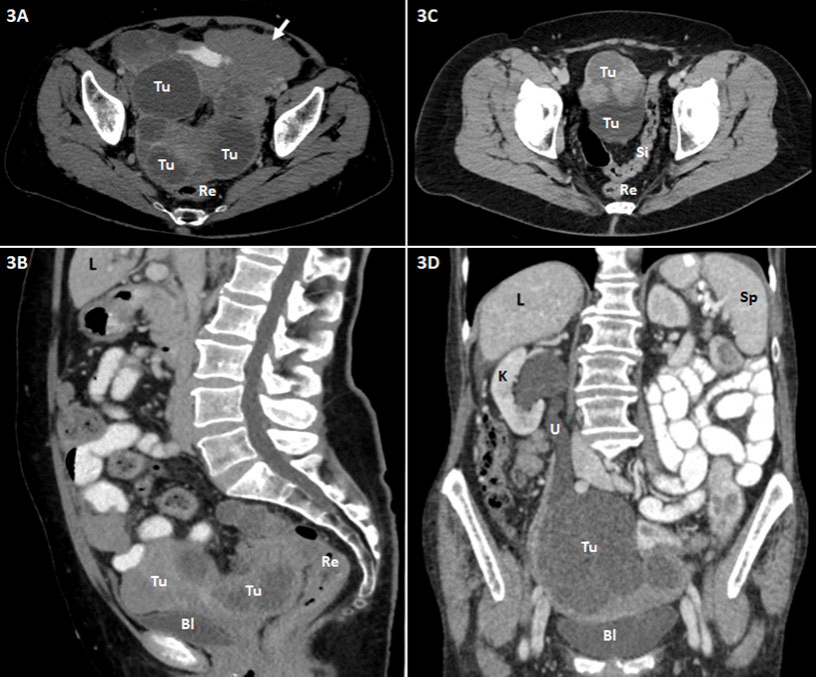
Abdominopelvic contrast-enhanced CT scans (A and C, axial images; B, sagittal reformatted image; D, coronal reformatted image) of patients with advanced ovarian carcinoma are shown. Bl, urinary bladder; L, liver; K, kidney; Re, rectum; Si, sigmoid colon; Sp, spleen; Tu, tumor (ovarian carcinoma); U, ureter. (A, B) Extensive tumor (Tu) in the pelvis consisting of large cystic and partly solid nodular parts. Close positional relationship and contact of the mass to the dorsally adjacent rectum (Re) and ventrally adjacent urinary bladder (Bl) with suspected rectal invasion and peritoneal involvement at the urinary bladder roof. Omental caking (short arrow) in the left side of the upper abdomen. (C) Massive tumor (Tu) in the pelvis including cystic and solid nodular parts. Close positional relationship and contact of the mass to the adjacent sigmoid colon (Si); tumor involvement of the latter is conceivable. (D) Large, predominantly cystic tumor mass (Tu) in the pelvis with involvement of the right ureter (U) and consecutive ipsilateral urinary retention and hydronephrosis of the right kidney (K).
